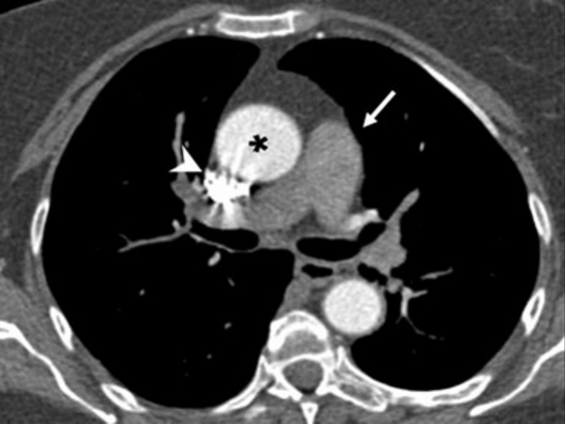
Transverse CT pulmonary angiogram at the level of the right pulmonary artery showing grade 1 transient interruption of contrast (TIC) in an 81-year-old woman. Pulmonary arteries (*arrow*) measure 72 HU, while the aorta (*asterisk*) is properly enhanced and contrast is still present in superior vena cava (*arrowhead*)
